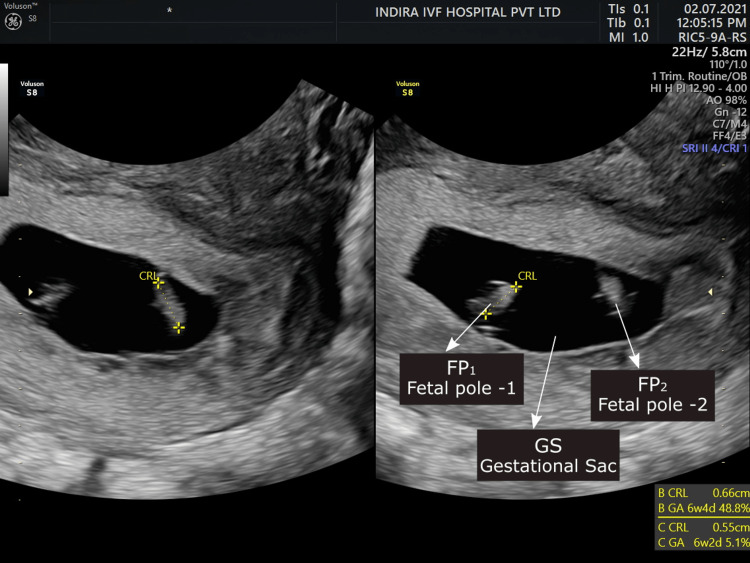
Ultrasound scan showing intrauterine live monochorionic twin pregnancy. FP1: Fetal pole 1; FP2: Fetal pole 2; GS: Gestational sac.
